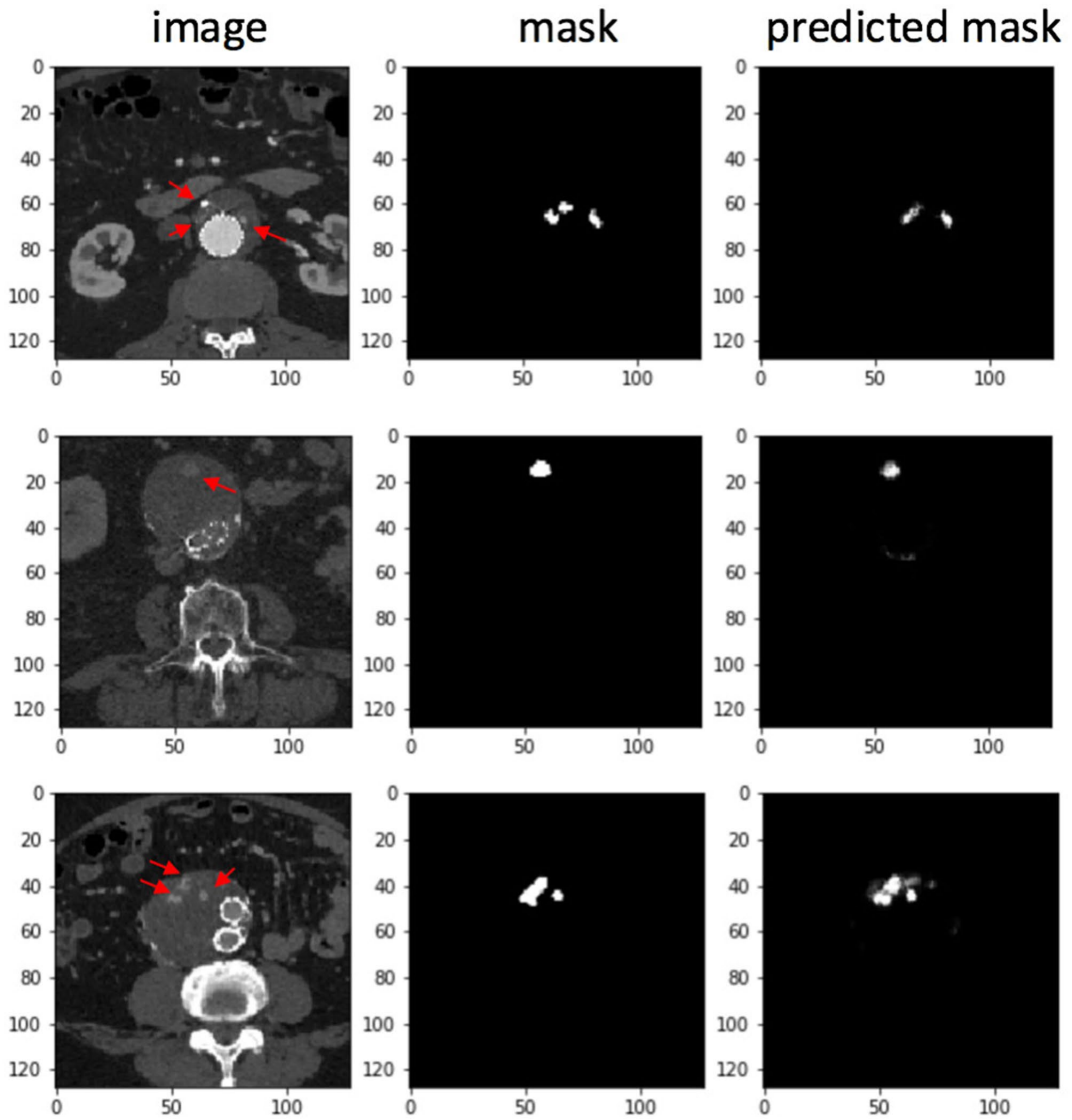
Raw CT slice (left) followed by human annotated segmentation map (middle) and model predicted endoleak map (right).
